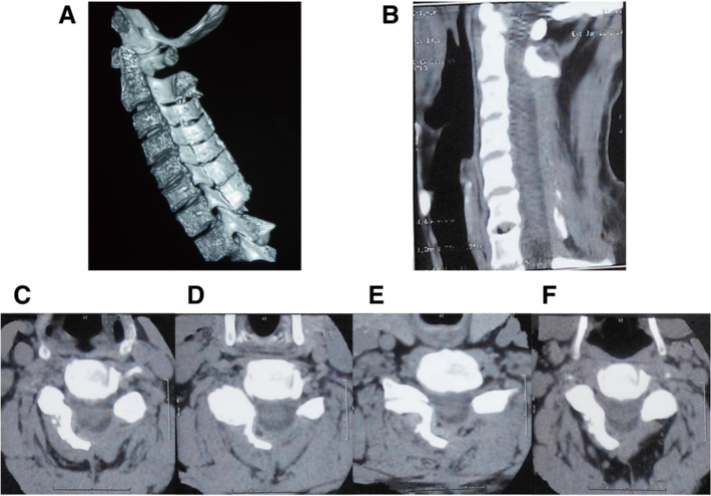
Representative CT images. A Three-D CT demonstrating expanded canal. Six-year postoperative 3-D CT with canal expansion maintained. B One-year postoperative sagittal CT image of a 62-year-old male. C–F Axial CT images of the case corresponding C3-4, C4-5, C5-6 and C6-7.
